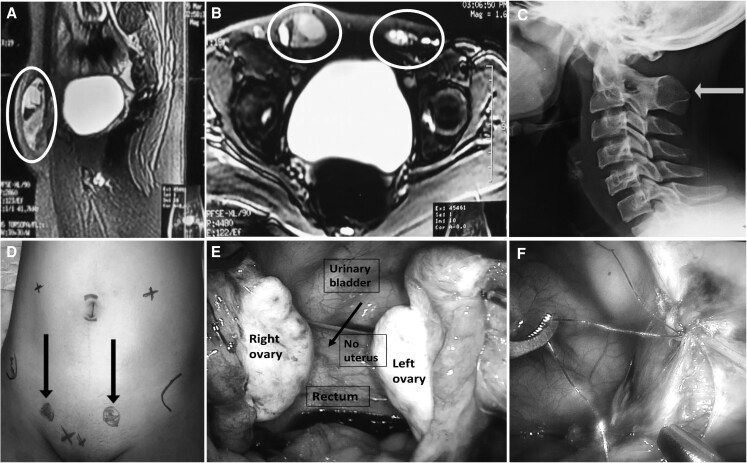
Imaging and intraoperative findings in case 2 with Müllerian agenesis and bilateral ovarian ectopia: (A) sagittal and (B) transverse sections of magnetic resonance imaging pelvis showing absence of uterus with ectopic edematous ovaries located in the parietal wall (white ovals); (C) lateral radiograph of cervical spine showing fusion of cervical vertebrae C2 and C3 (white arrow); (D) intraoperative image showing marking of the positions of ectopic ovaries (black arrows) and laparoscopic ports (cross marks); (E) both ovaries after reduction into the abdomen; (F) inguinal canal repair.
